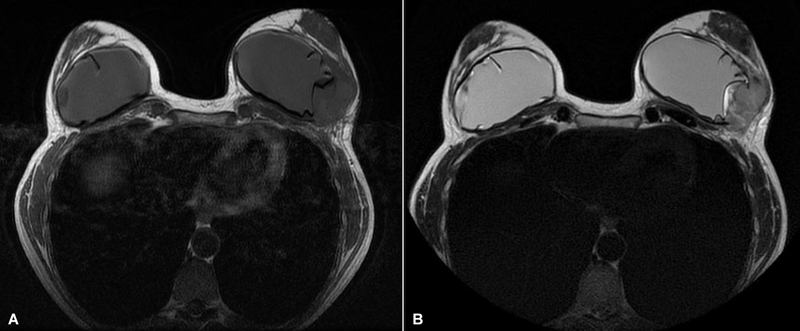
Axial images from a breast MRI. (
